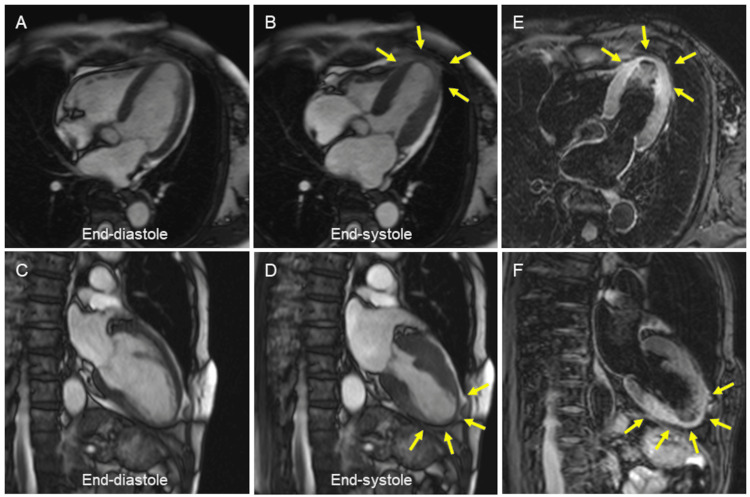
Cardiac MRI Cine MRI revealed regional wall motion abnormality in the LV apex (A to D, arrows), where T2-weighted MRI detected myocardial edema, indicative of the myocardial area at risk (E and F, arrows). LV, left ventricular; MRI, cardiac magnetic resonance imaging
